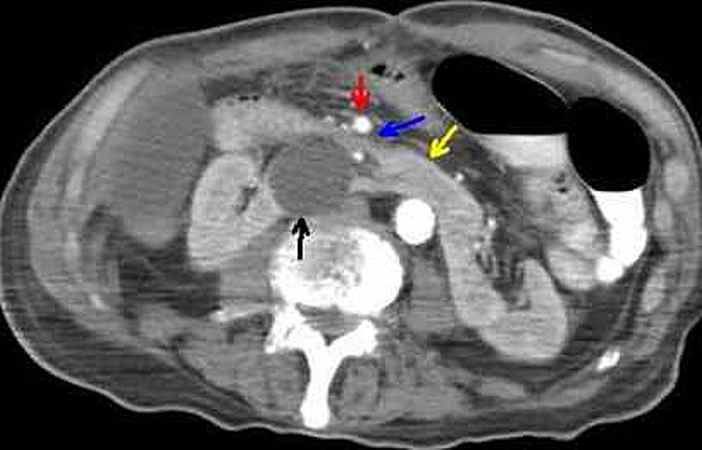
Review of CT images revealed duodenal dilation proximal to the level of the 3rd part (blue arrow) which was compressed by the close proximity of superior mesenteric artery (red arrow) to the renal cyst (black arrow) and isthmus (yellow arrow) of the horseshoe kidney. Aortomesenteric angle and distance had values above normal
